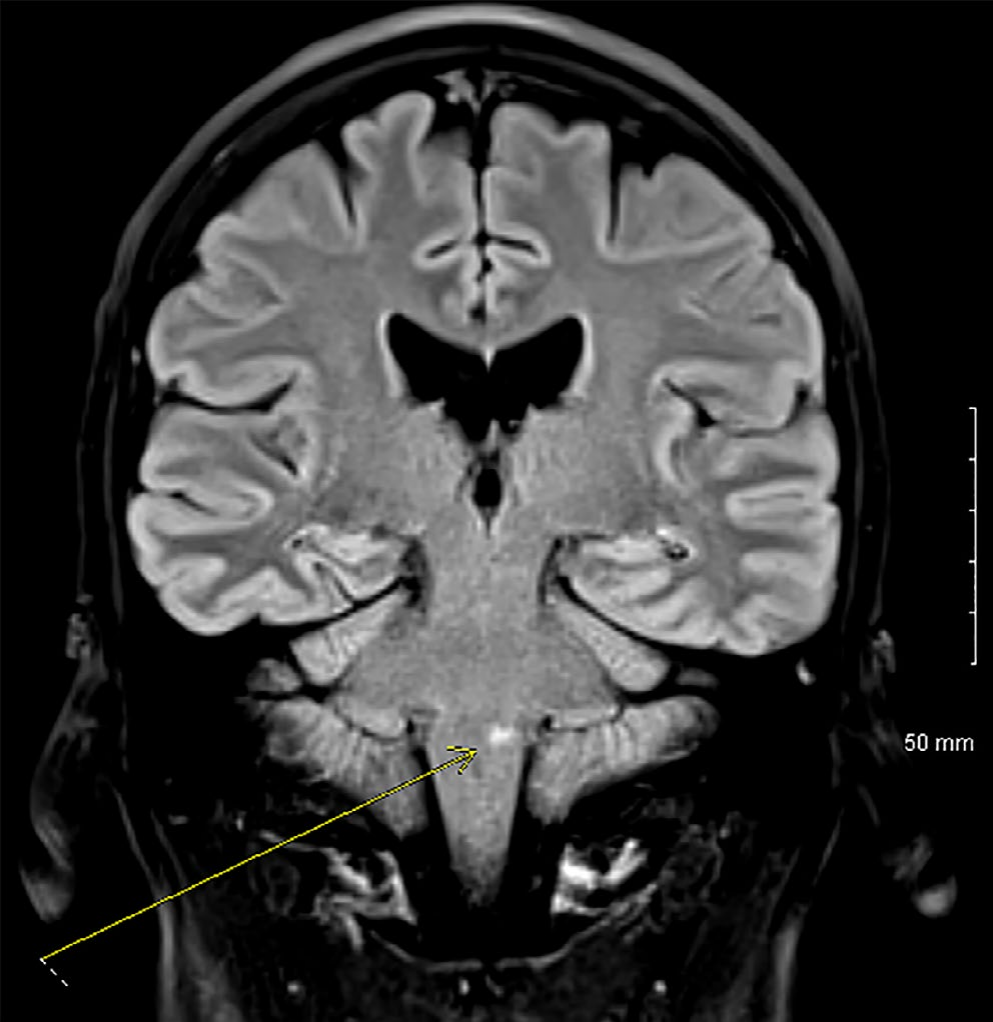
Coronal image showing T2 FLAIR hyperintensity of the left medullary region (yellow arrow)
